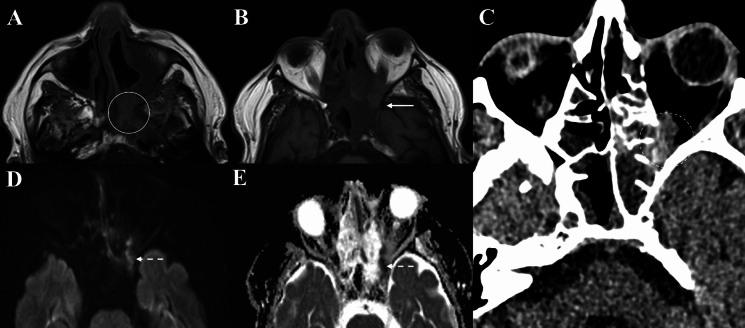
Orbital imaging of a 69-year-old male with invasive aspergillosis of the left orbit. A and B Demonstrates obliteration of fat planes and an infiltrative mass in the left retro-antral tissues involving the left orbital apex and causing proptosis on axial T1 sequences (Solid circle and arrow). This mass is slightly T1-hyperintense. C Depicts bony erosion and hyperdense fungal material within the left ethmoid sinus and orbital apex on axial unenhanced CT (Dashed circle). D and E Demonstrates regions of diffusion restriction within the left ethmoid sinus and orbital apex corresponding to the area of fungal infection (Dashed arrows)
